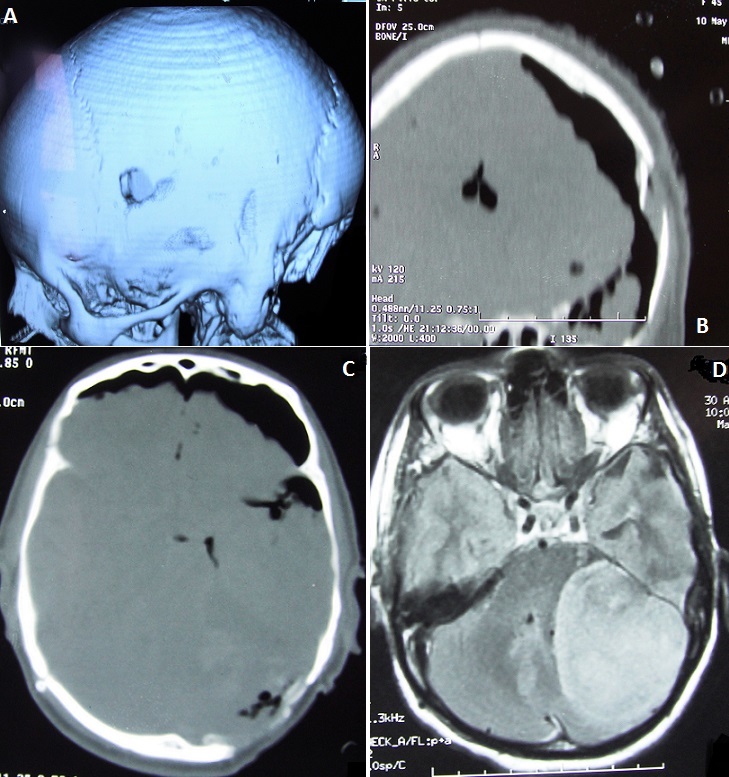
Postoperative computed tomography scan head showing left frontal depressed fracture (A, B, C) with significant pneumocephalus; (B): axial(D) T1-weighted view of magnetic resonance imaging scan demonstrate meningioma in the left posterior fossa
